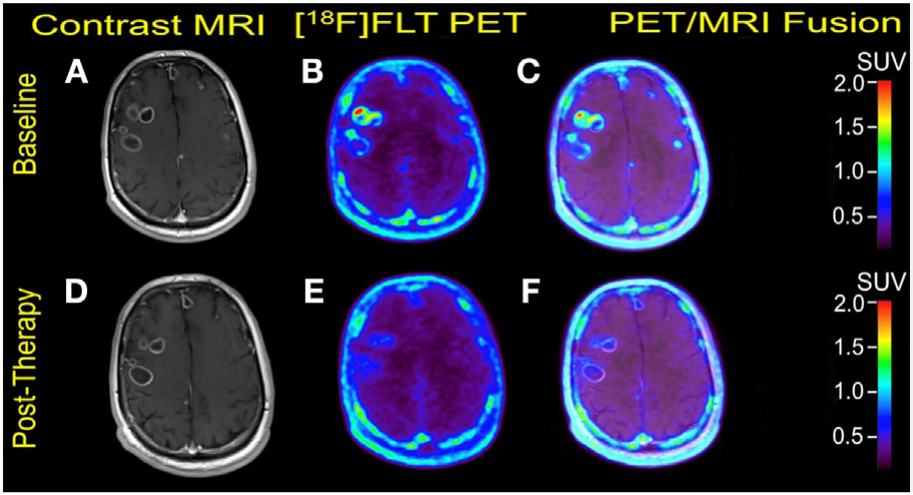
Case #1. Baseline magnetic resonance imaging (MRI) (A), fluorothymidine (FLT)–positron-emission tomography (PET) (B), and fused PET/MRI (C); follow-up MRI (D), FLT–PET (E), and fused PET/MRI (F) at 3 weeks. Bilateral supratentorial brain lesions of greater than 1 cm demonstrate variable mild to intense FLT avidity. They showed a greater proliferative reduction compared with the size reduction at 1-month follow-up. Gadolinium-enhanced T1-weighted spin echo with TR 708 ms, TE 8.4 ms, and 90° flip angle, 5 mm slice thickness.
